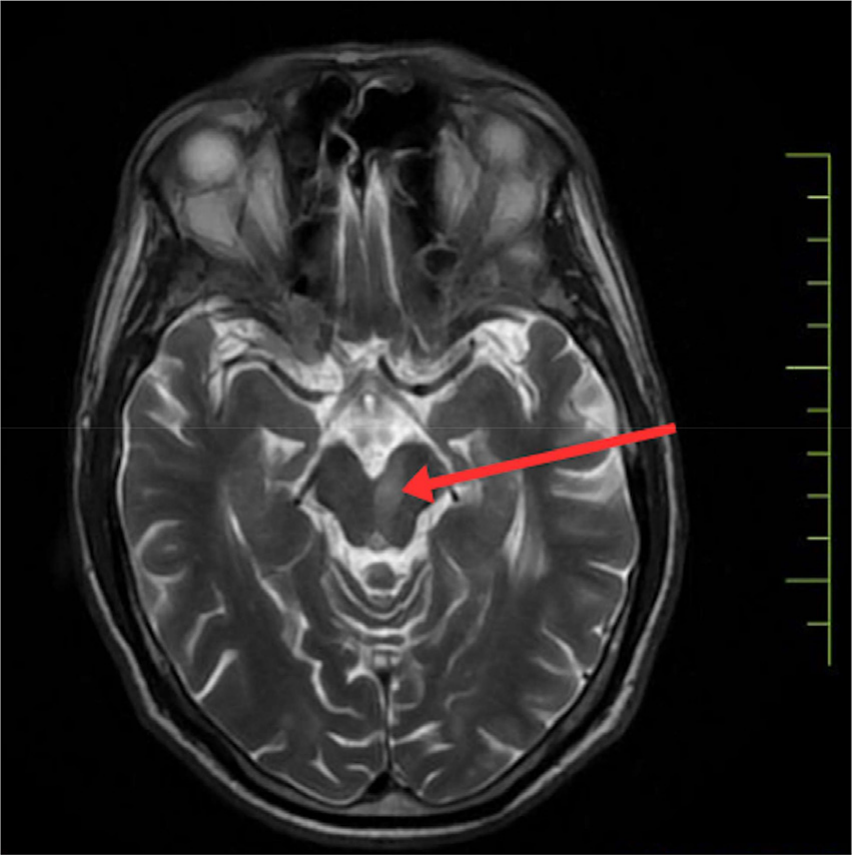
Shows the hyperintensity in the left paramedian midbrain on the patient's axial T2 section of the MRI.
